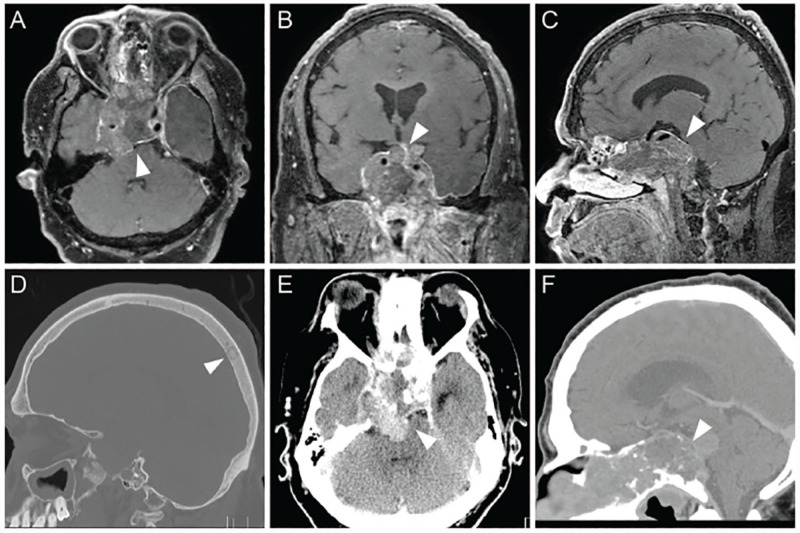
Patient transition to pituitary carcinoma Gadolinium-contrasted T1 brain MR images in axial (A), coronal (B) and sagittal (C) planes demonstrating enlarging invasive macroadenoma (arrowheads). Sagittal non-contrasted head CT in bone window reveals sclerotic calvarial lesion (arrowhead) (D). Non-contrasted head CT imaging revealing continued invasive growth of the pituitary adenoma around the clivus and prepontine cistern, precipitating marked brainstem compression, axial (E) and sagittal (F) (arrowheads).
